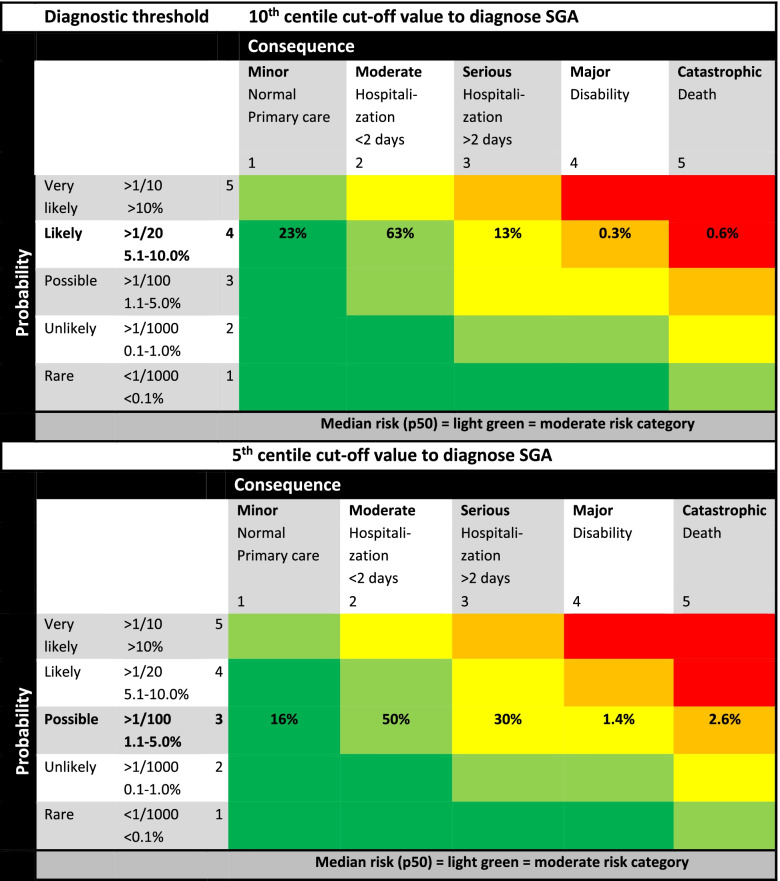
Risk matrix approach displaying the differences in neonatal consequences for small for gestational age fetuses, using the 10th and 5th centile as threshold for the fetal abdominal circumference measured by ultrasound [15]
